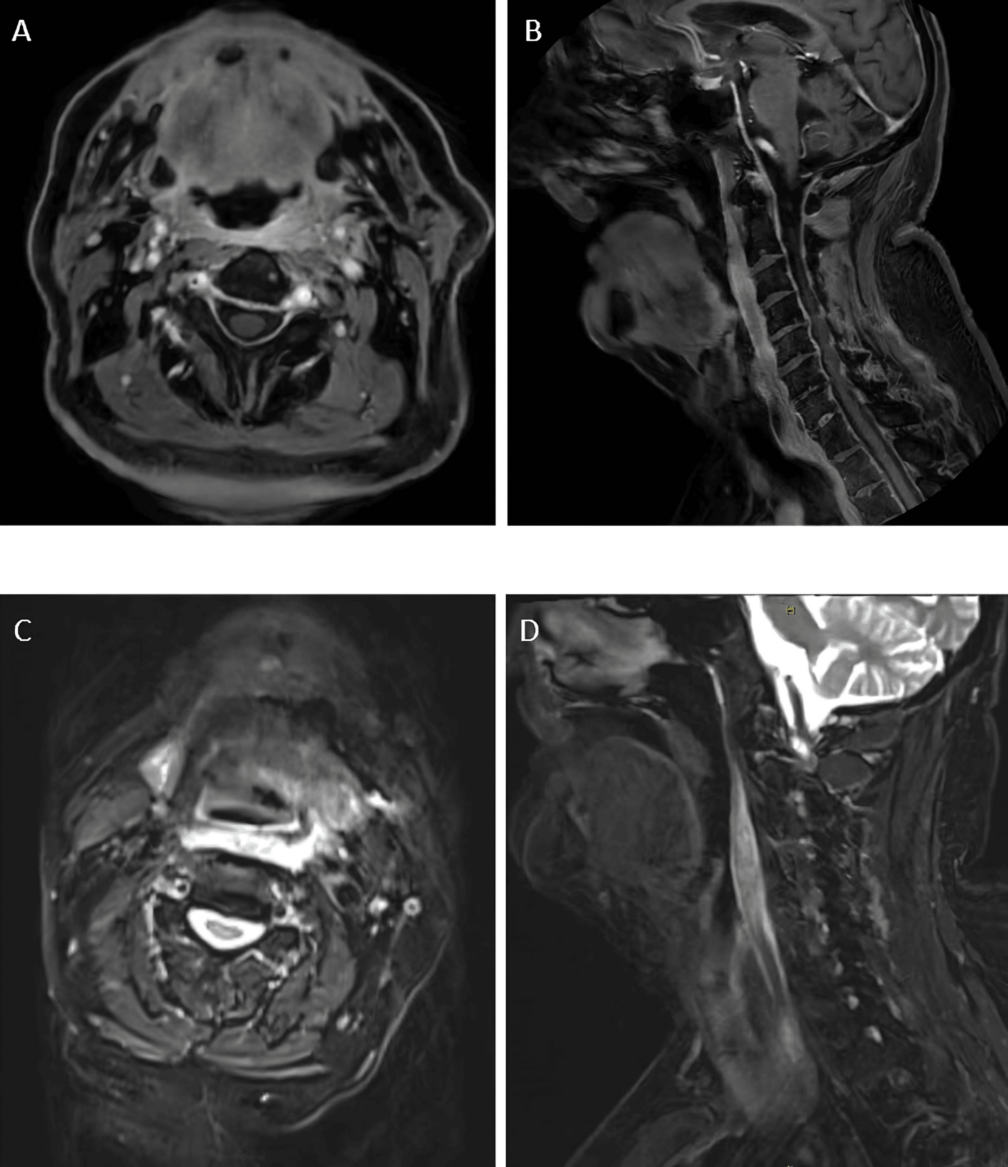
Multi-sequence, multiplanar gadolinium-enhanced images demonstrating inflammatory changes in the left retropharyngeal space with radiological features suggestive of longus colli calcific tendonitis. (A, B) are T1-weighted images showing contrast enhancement in the left retropharyngeal and prevertebral spaces in the axial and sagittal planes, respectively, while (C, D) show concordant edema on T2-weighted sequences.
